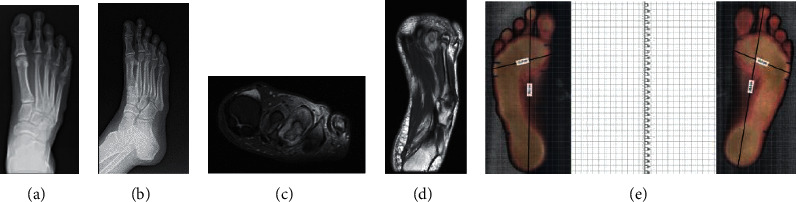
(a–e) Results of imaging tests (X-ray, MRI) and foot scanning: patient no. 10. (a) Dorsal-plantar X-ray: degradation lesion of the III head of the metatarsal bone and numerous transverse calcifications. (b) Diagonal X-ray of foot 45: degradation lesion of the III head of metatarsal bone and numerous calcifications. (c) Magnetic resonance imaging with contrast: degradation lesion of the III head of the metatarsal bones and medial plantar nerve neuralgia. (d) Magnetic resonance imaging with contrast: degradation lesion of the III head of the metatarsal bone and medial plantar nerve neuralgia. (e) Plantar photo from 2D Scanner: assessment of plantar part of the foot and measurement of foot length and width.
